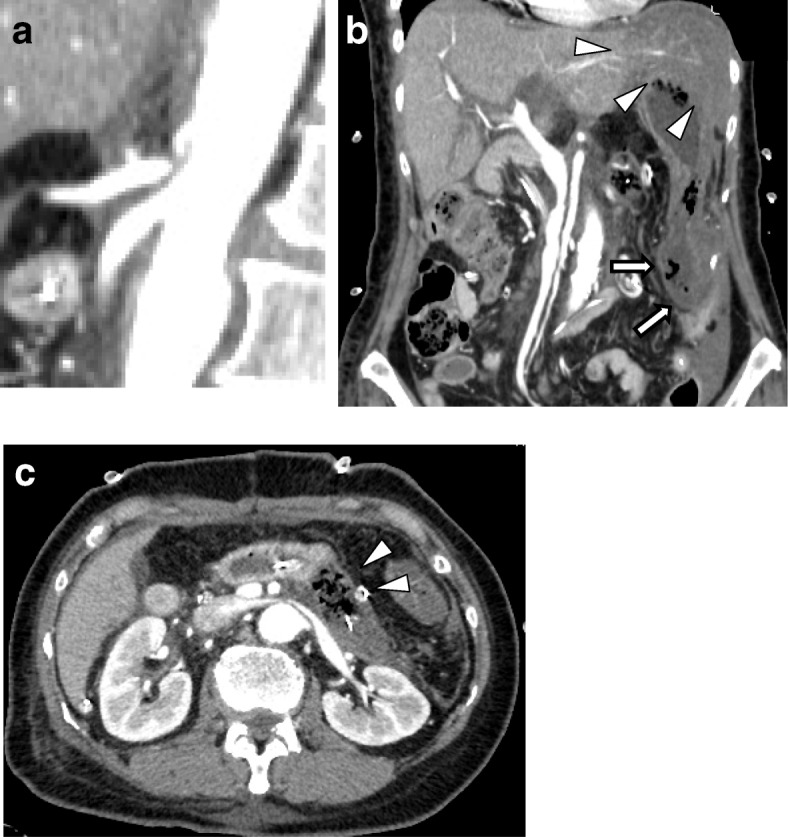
Contrast-enhanced multidetector CT at POD 3 showing an acute extrinsic stenosis caused by median arcuate ligament compression (a), ischemia in the liver (arrow head) and the gastrojejunostomy site (arrow) (b), and necrosis of the pancreatic body (c)
